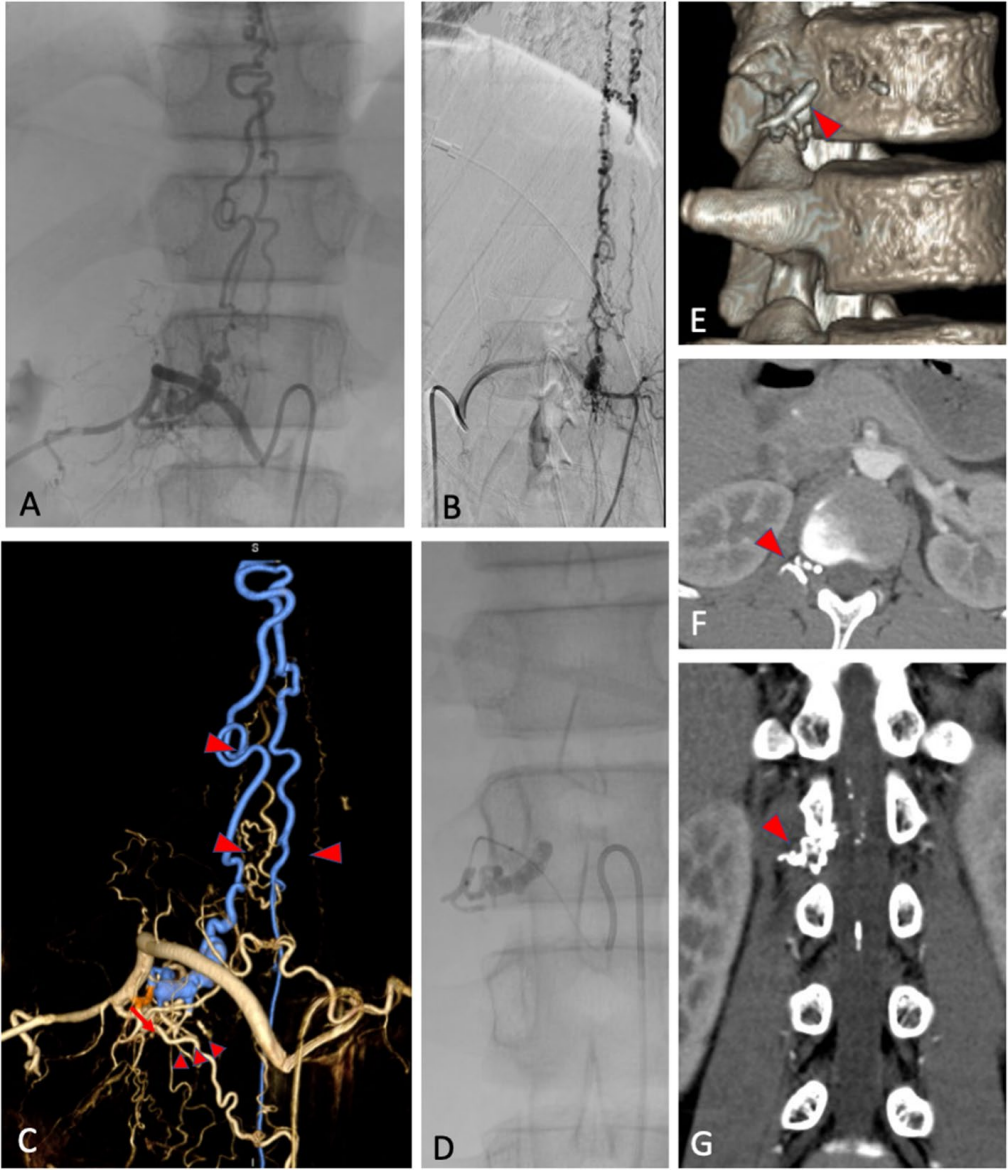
Antero-posterior (A) and lateral (B) views and three-dimensional reconstruction angiogram (C) of the SDAVF from right L2 segmental artery demonstrated the fistula (arrow) was fed by radicular arteries and drained retrogradely through an arterialized radicular vein (small arrowheads) into perimedullary veins (large arrowheads). The n-butyl cyanoacrylate (NBCA) occluded the radicular arteries and proximal draining vein (D). 3D reconstruction (E), axial (F), and axial view (G) of the computed tomogram demonstrated the NBCA casting (arrowheads) around the tumor at the right L1/L2 intervertebral foramen
